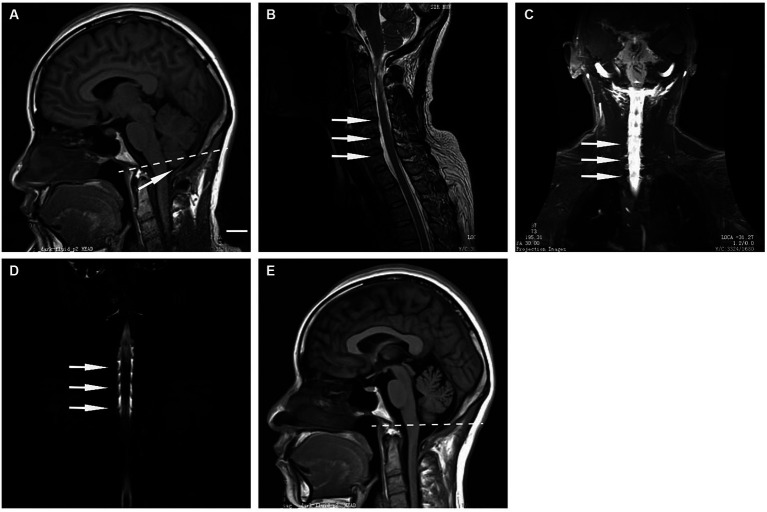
(A) Sagittal T1-weighted MRI performed at 1 month after the onset of headaches showing 5 mm prolapse of the cerebellar tonsils (arrow) into the foramen magnum (dashed line). (B) Sagittal T2-weighted MRI showed a crowded spinal canal. (C) Intrathecal gadolinium-enhanced MR myelography showed CSF leakage in the cervico-thoracic junction. (D) MR myelography indicated that the CSF leakage site was located in the cervico-thoracic junction. (E) Sagittal T1-weighted MRI performed at 2 months after targeted EBP intervention showed that re-ascent of the cerebellar tonsils above the foramen magnum (dashed line). Scale bar: 1 cm.
